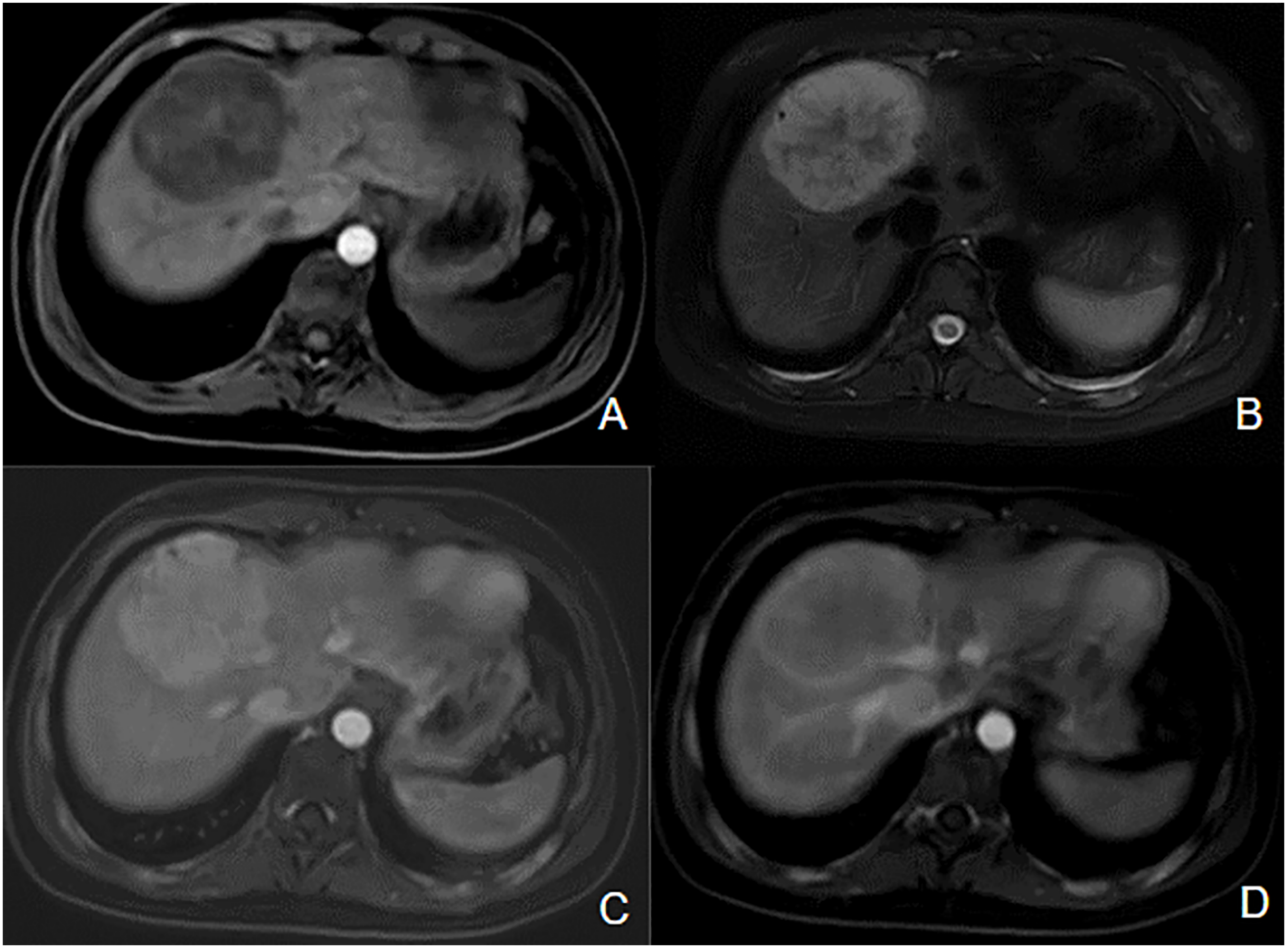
Inflammatory hepatocellular adenoma in a patient with MAS. Hypointense mass located on the junction of segments VIII and IV of the liver on axial T1-wieghted image (A), hyperintense on T2-weighted image (B). We can observe enhancement on the T1-postcontrast arterial phase image (C), persistent during the portal phase (D).
